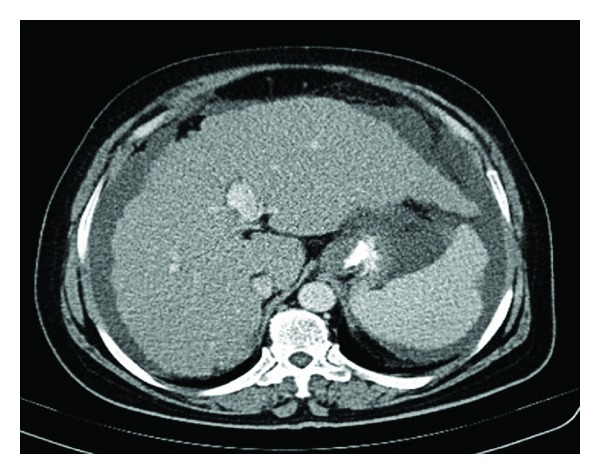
Abdominal CT scan showed a cirrhotic liver, splenomegaly, and ascites.
